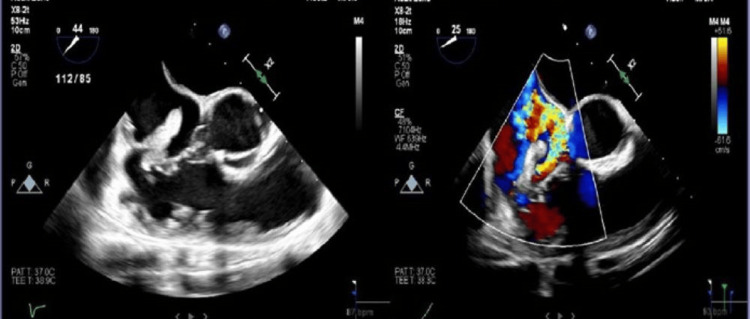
(Left and right) Transesophageal echocardiogram findings of marked thickening of tricuspid leaflets, and large pedunculated, solid, highly mobile vegetation around 2 cm on the body of the septal leaflet with very severe tricuspid regurgitation
